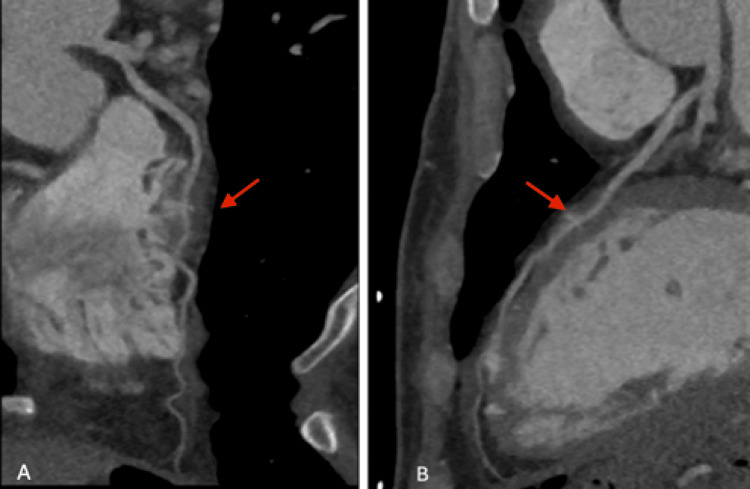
Coronary computed tomography angiography. A and B: Angiography showing myocardial bridging (arrow) of the left anterior descending coronary artery (LAD).
